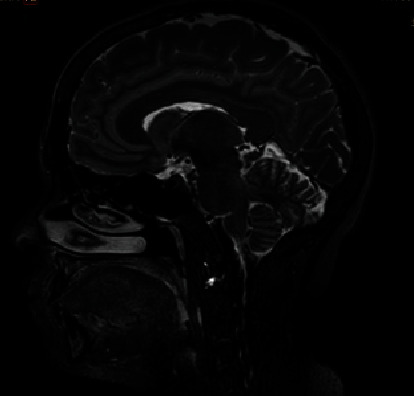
MRI of the pituitary gland, which is rather small for age and sex but without evidence of focal abnormalities.
